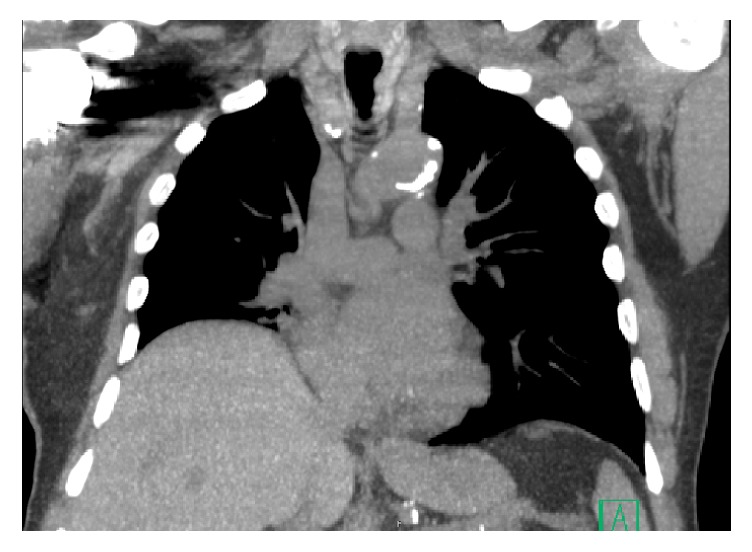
Chest CT scan 6 weeks postoperatively.
